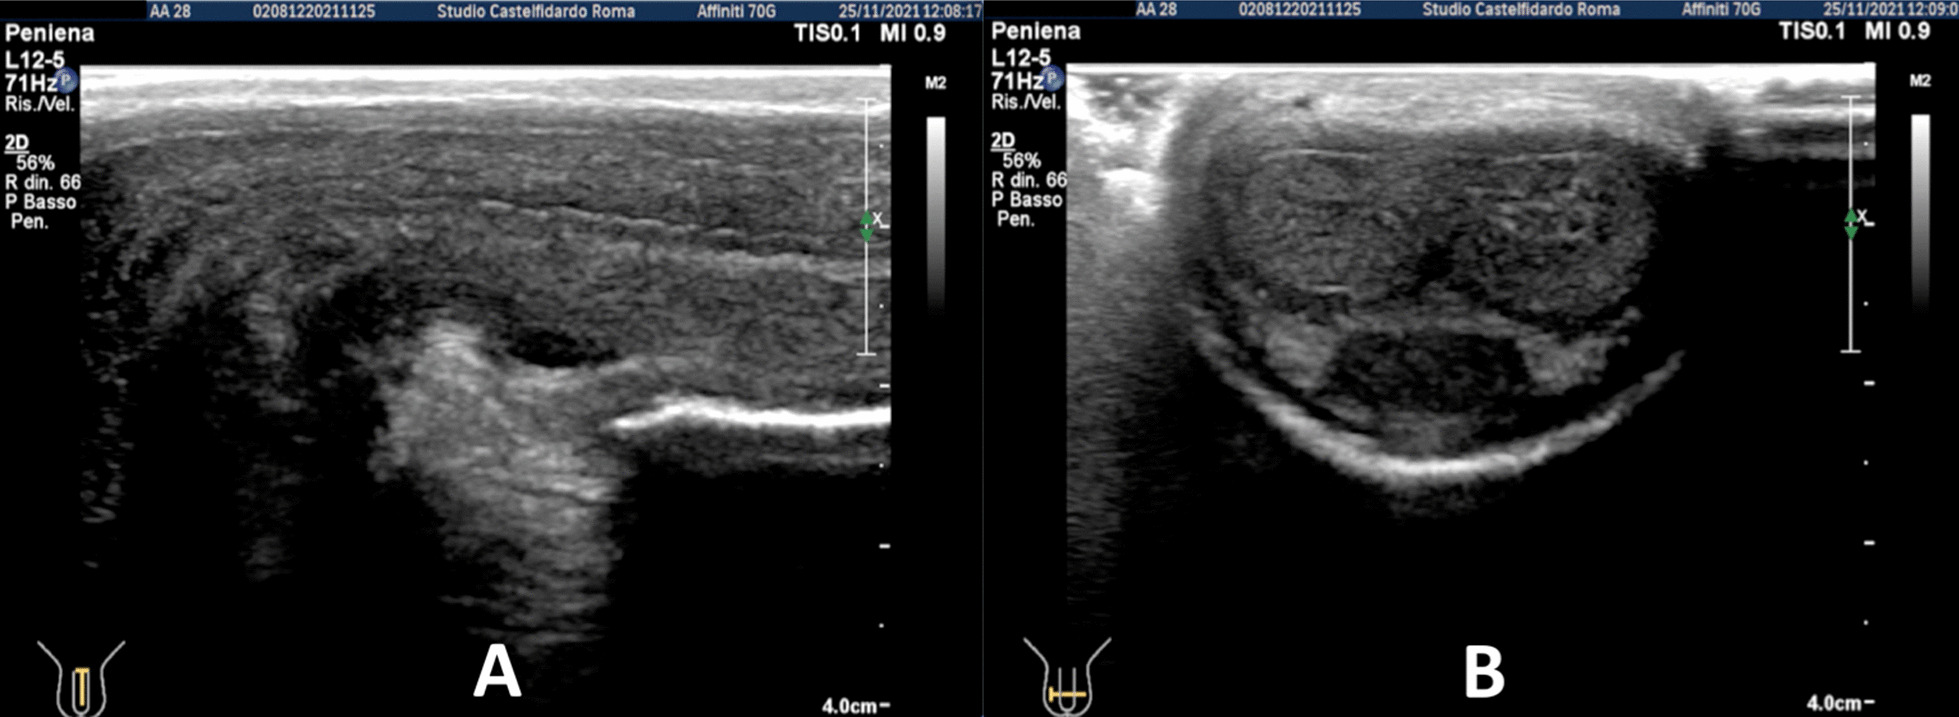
Penile ultrasound after the second treatment cycle. A longitudinal scan. B Transverse scan
